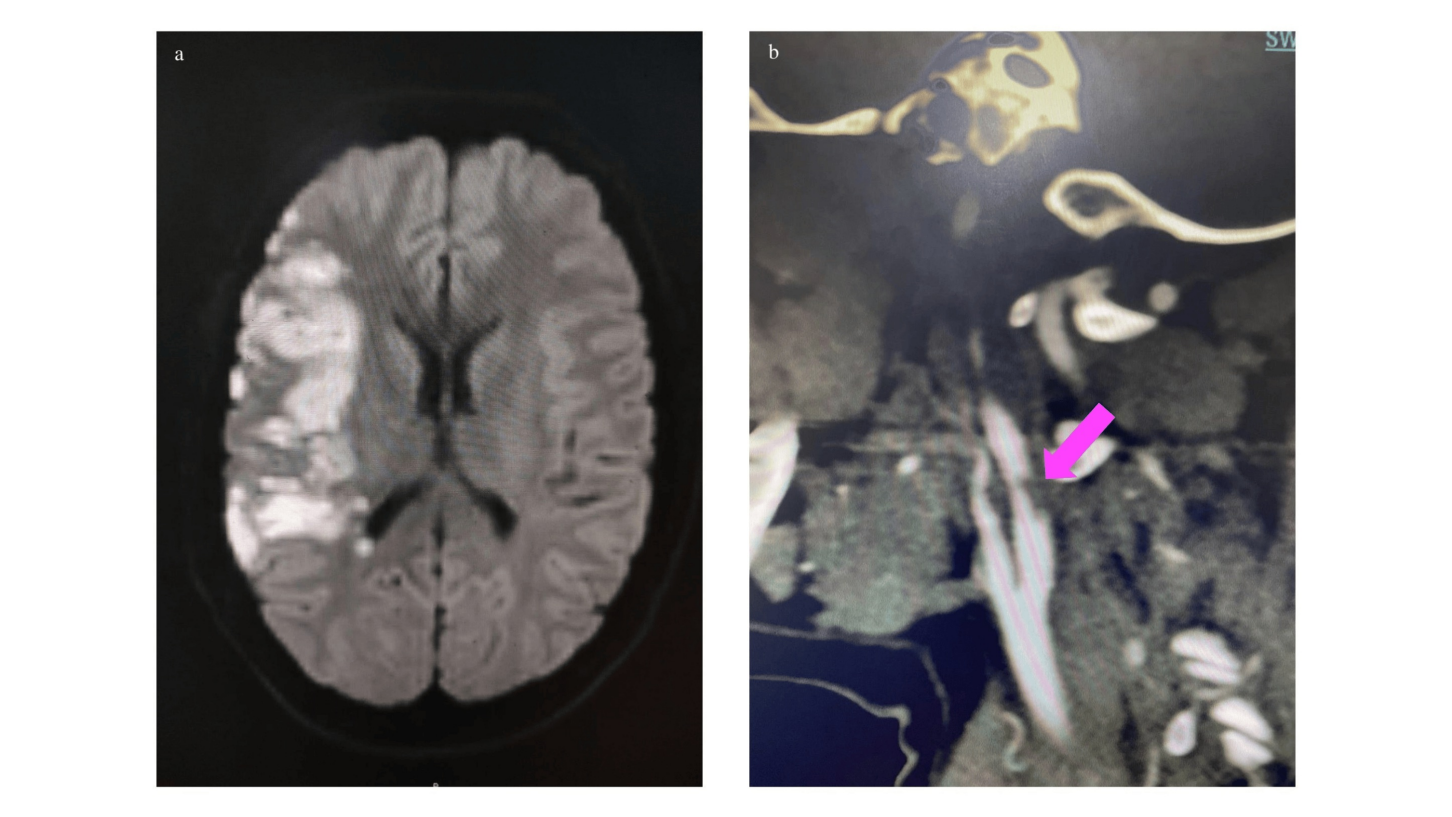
a. MRI of the brain (DWI) showing an acute infarct in the right MCA territory; b. CT angiography showing a right ICA ulcerated plaque (pink arrow). MRI, magnetic resonance imaging; DWI, diffusion-weighted imaging; MCA, middle cerebral artery; CT, computed tomography; ICA, internal carotid artery
